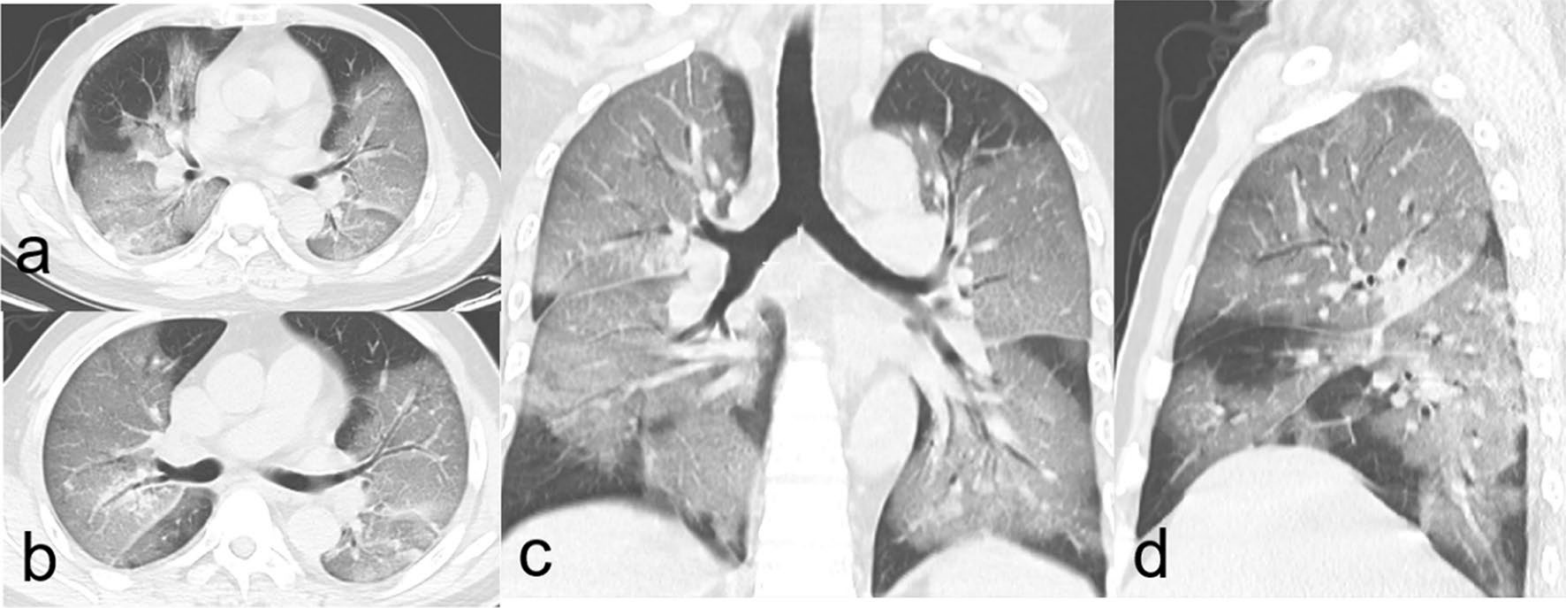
Thin-section CT scans in a man infected with SARS-CoV-2. Scan obtained on 7th day from onset of symptoms shows diffuse ground glass opacities that affected bilateral lung parenchyma. The patient died 10 days after this scan due to respiratory failure.
